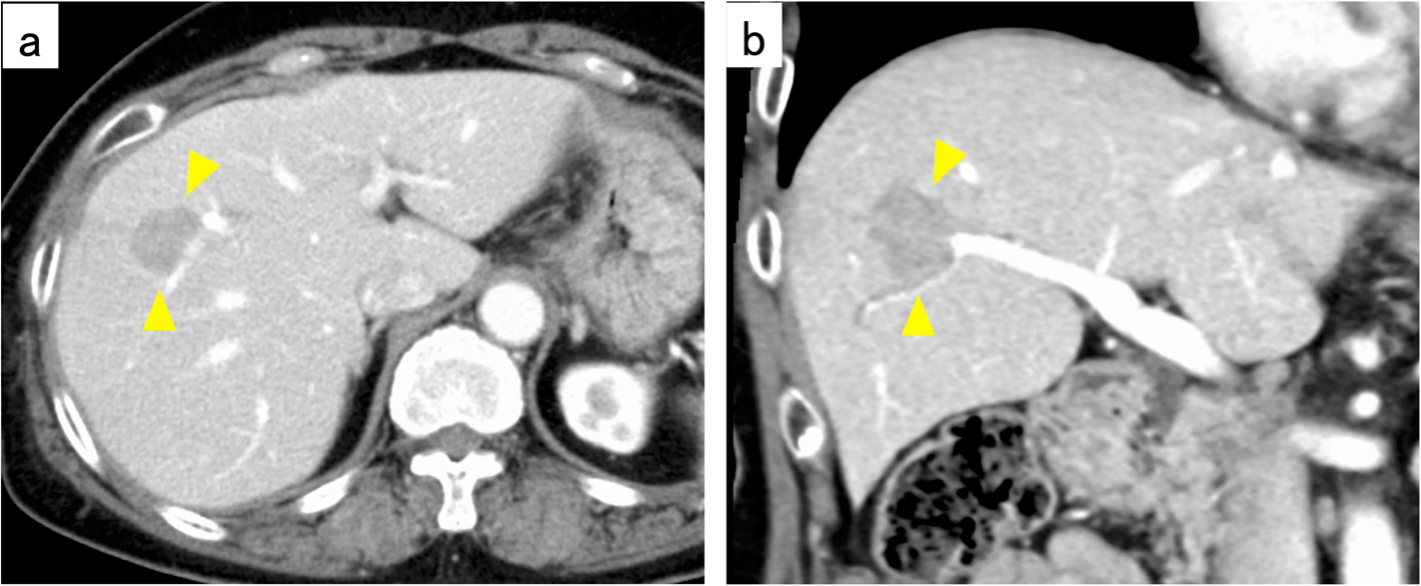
Contrast-enhanced CT scan revealed a tumor measuring 2.5 cm in diameter at Couinaud segment V of the liver (a Axial, b Coronal)
